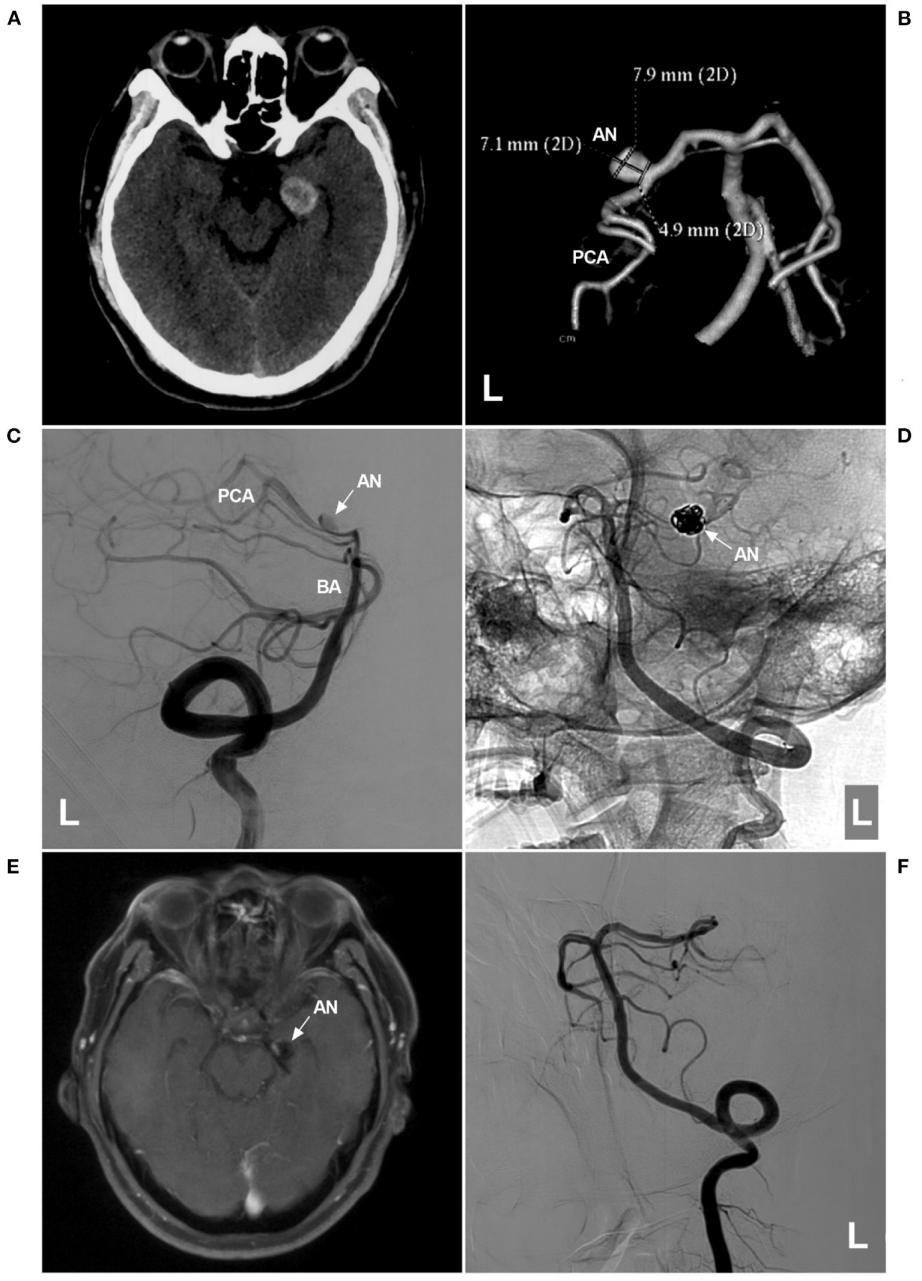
Assisted coiling of P2 segment aneurysm with thrombosis. (A) CT shows a highly dense lesion beside the left midbrain. (B) CTA showing a dissecting aneurysm (AN) on the P2 segment. (C,D) Two-dimensional angiograms of the left VA show the preoperative (C) and postoperative (D) images. In D, the coils (arrow in D) in the aneurysm sac were larger than the aneurysm shape in C, indicating thrombosis in the aneurysm. (E) One-year follow-up MRI showing that the thrombus was absorbed, and the aneurysm (arrow and AN) was smaller than that in A. (F) One-year follow-up angiogram showing no recurrence of the aneurysm. AN, aneurysm; BA, basilar artery; CT, computed tomography; CTA, CT angiography; L, left; MRI, magnetic resonance imaging; PCA, posterior cerebellar artery; VA, vertebral artery.
